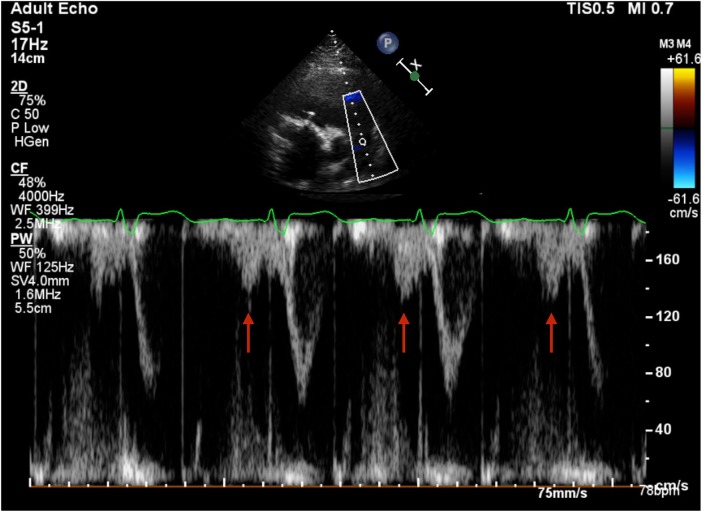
Pulse wave Doppler at the right ventricular (RV) outflow tract in short
